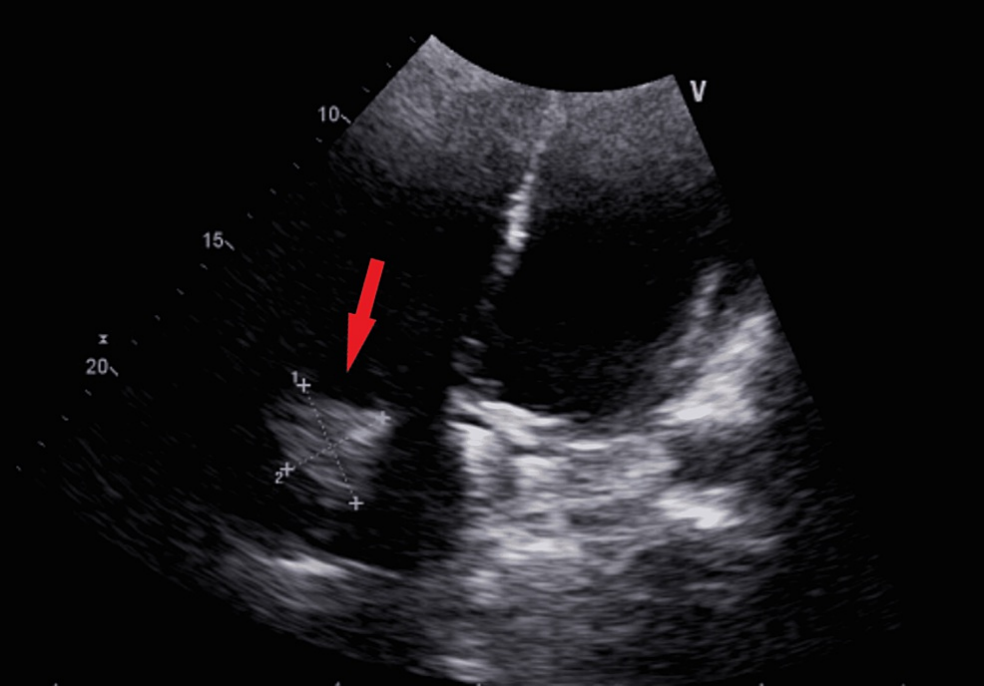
Transthoracic echocardiography showing a 3.8 cm
